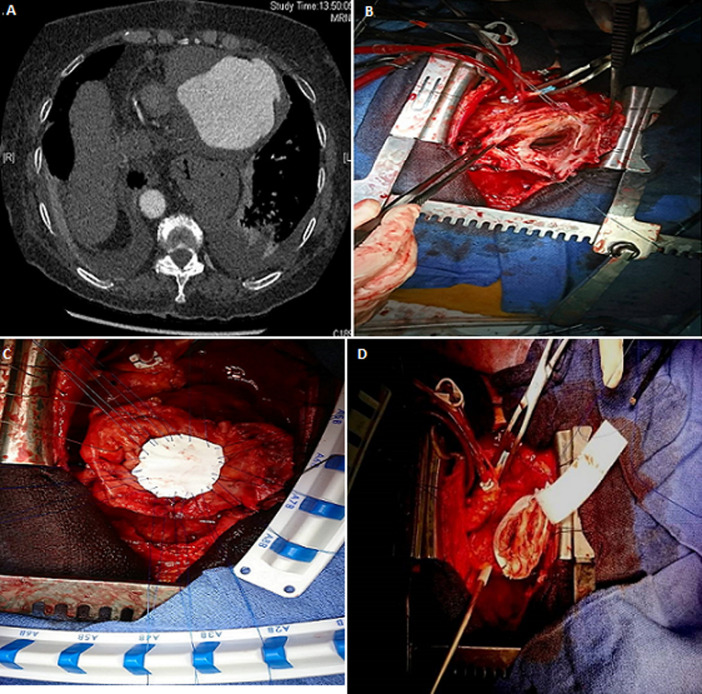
A) computed tomography showing a huge apical pseudoaneurysm of the left ventricle with extravasation of the radiocontrast agent in the pericardium; B) a perioperative view showing the apical pseudoaneurysm of the left ventricle; C) a perioperative view showing the closure of the defect by a Gore Tex patch; D) a perioperative view showing the closure of the remaining re-entries by Teflon felt support
